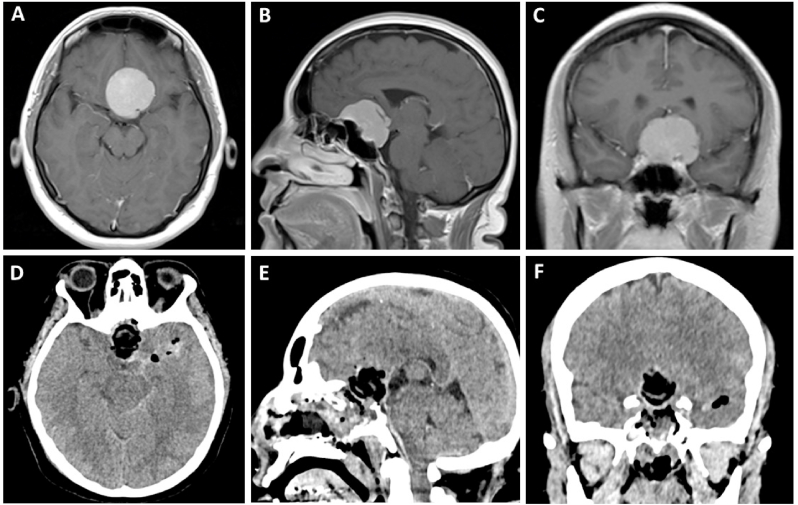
Preoperative MRI: (A) axial, (B) sagittal, and (C) coronal views from a T1WI C+ revealed an homogeneous enhancing solid sellar lesion, originating from the tuberculum sellae with extension to the sellar region and upper third of the clivus. Postoperative CT scan: (D) axial, (E) sagittal, and (F) coronal views in which gross total resection is observed.
